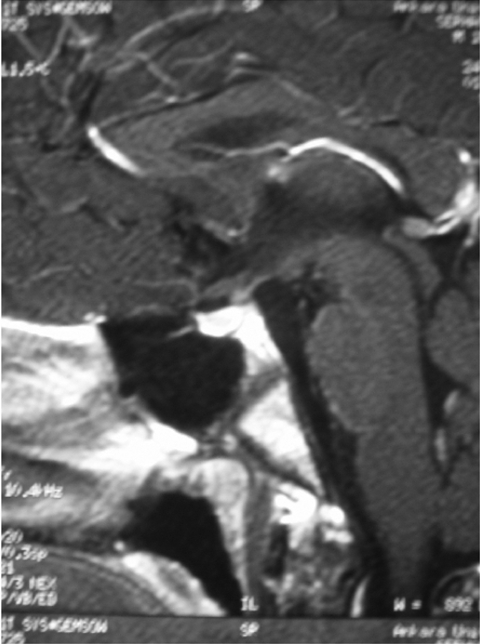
MRI at follow−up: Regression of the PST from 7 mm to 2.5 mm on MRI
